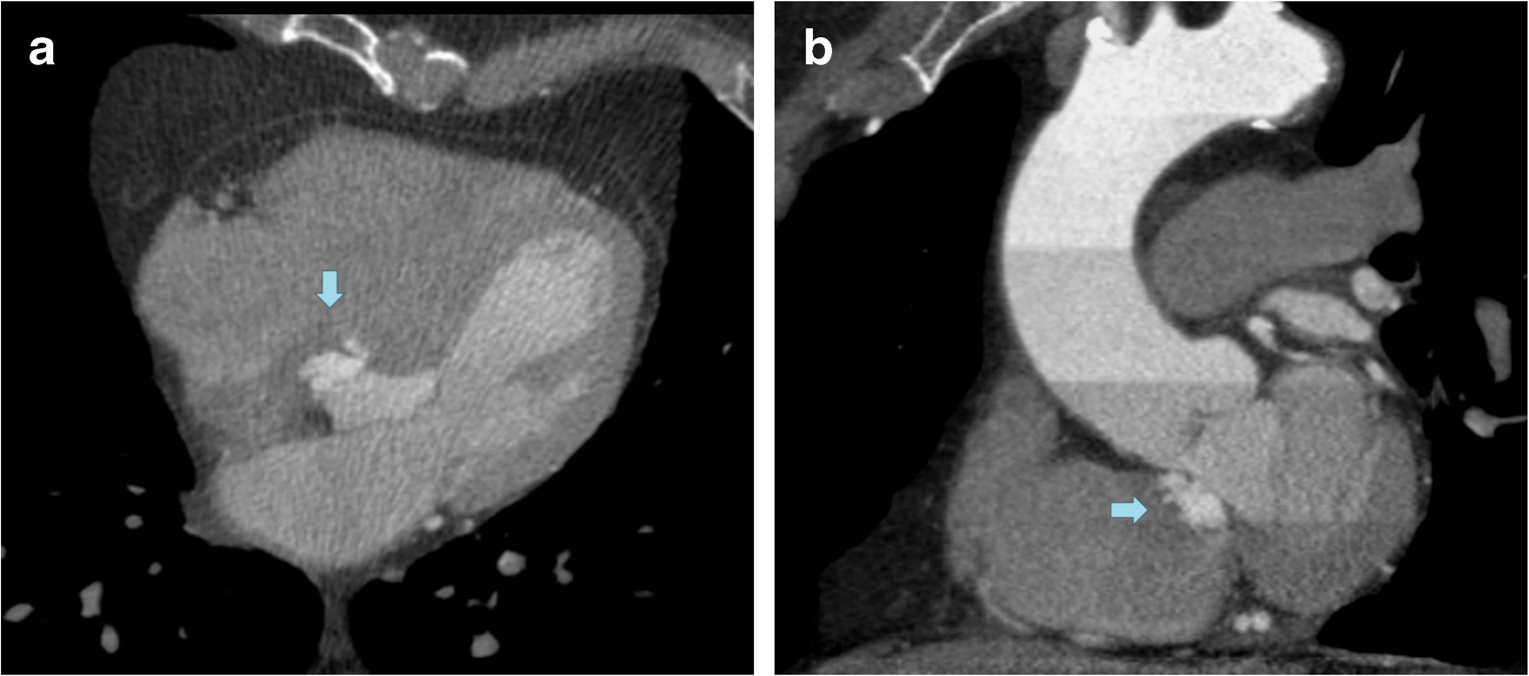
78-year-old man with right heart failure was diagnosed with IVMS aneurysm in the evaluation of cardiac CT. CT axial view and coronal oblique MPR (a and b) show a small lobulated out-pouching of the membranous portion of the IVMS (*arrows*) extending into the right ventricle
